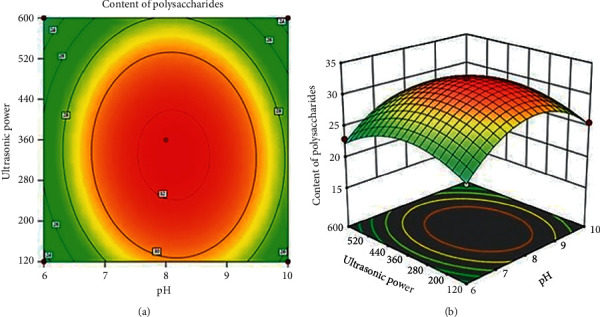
Simultaneous effect of pH and ultrasonic power on the content of polysaccharides.
